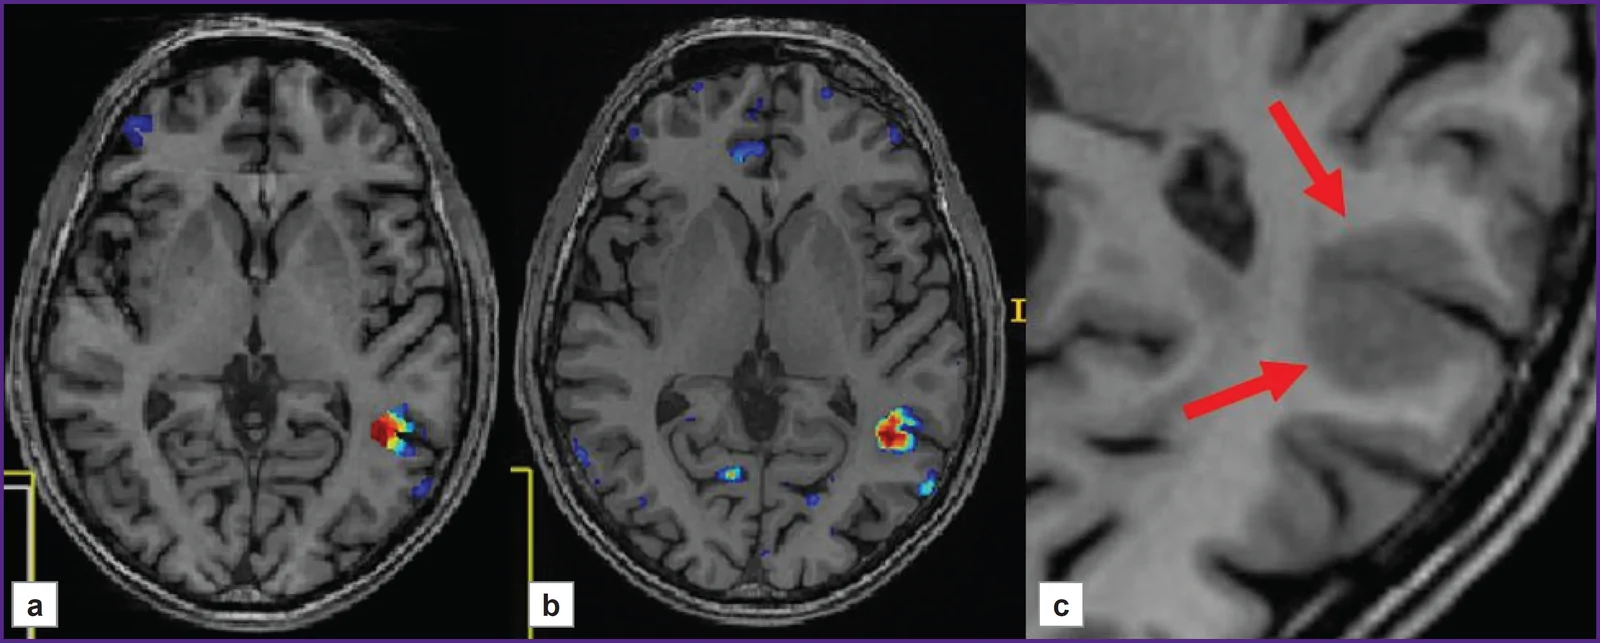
MRI scan of a 50-year-old man with focal cortical dysplasia in the left temporoparietal region: (a) classical algorithm: cortical thickening (red area); (b) multimodal algorithm: confirmation of cortical thickening (red area); (c) T1 images showing the cortical thickening in the left temporo-parietal region, with the arrows indicating the area of change
